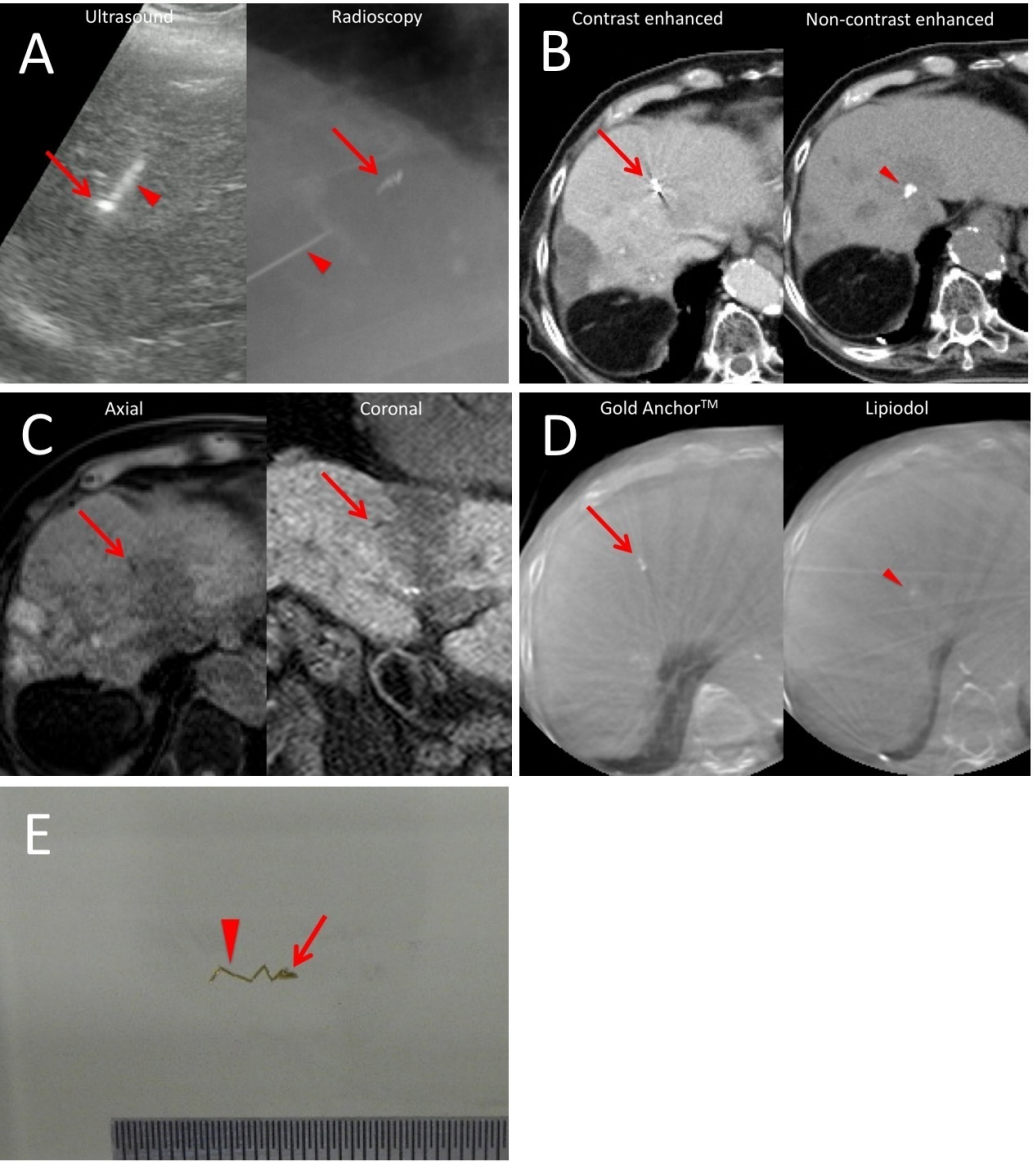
Placement of the GA; the GA in radiographic images. These images show the placement of the Gold Anchor^TM ^(GA) in an 81-year old man with recurrent hepatocellular carcinoma after 11 rounds of radiofrequency ablation and eight rounds of transcatheter arterial chemoembolization. The Gold Anchor^TM^ (GA) was percutaneously injected into the liver close to the tumor under ultrasound and radiographic guidance (A). The arrow and arrowhead indicate the GA and the needle, respectively. The GA showed the tadpole-like shape. The GA was well-detected in both images on computed tomography (CT) (B, left) and gadolinium-ethoxybenzyl-diethylenetriamine pentaacetic acid (Gd-EOB-DTPA)-enhanced magnetic resonance imaging (MRI) (C). The uptake of lipiodol emulsion was also well-detected on CT (B, right, arrowhead). In cone-beam CT (CB-CT) for image-guided radiotherapy (IGRT), the GA (arrow) showed a better detectability than the uptake of lipiodol emulsion (arrowhead) (D). The tadpole-like shape of the GA (E). A folded shape (arrow) followed by a linear shape (arrowhead). GA = Gold Anchor^TM^
